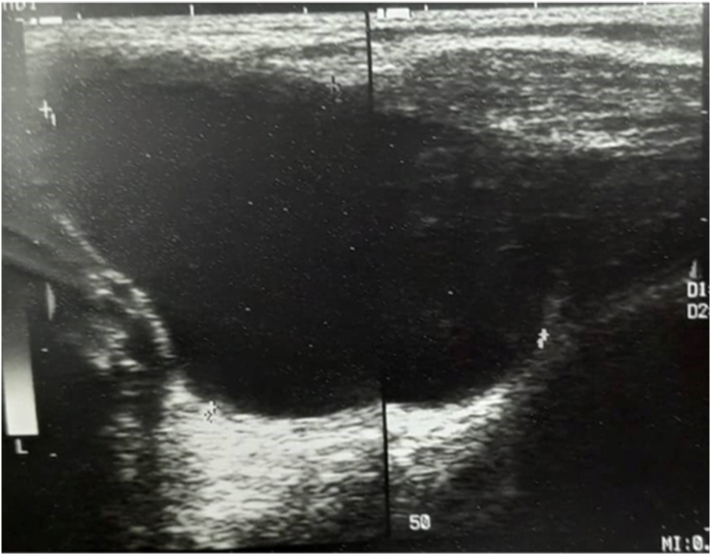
High linear frequency (10–15 MHz) ultrasound probe on a transverse cranial scan of the right axilla neck with a colour-Doppler module shows a hypoechoic unilocular cystic lesion with an absence of identifiable flow signal.
